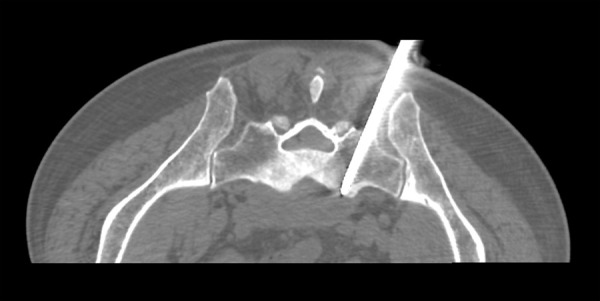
Transverse CT image showing bone marrow biopsy needle penetrating the sacrum.
